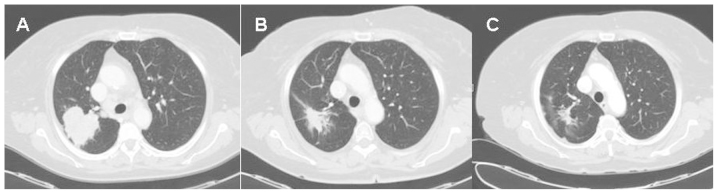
Case 1: (A) CT scan showing a lesion in the upper lobe of the right lung, (B) CT scan after three months of chemotherapy indicated a partial response and (C) CT scan showing complete response after six cycles of chemotherapy. CT, computed tomography.
